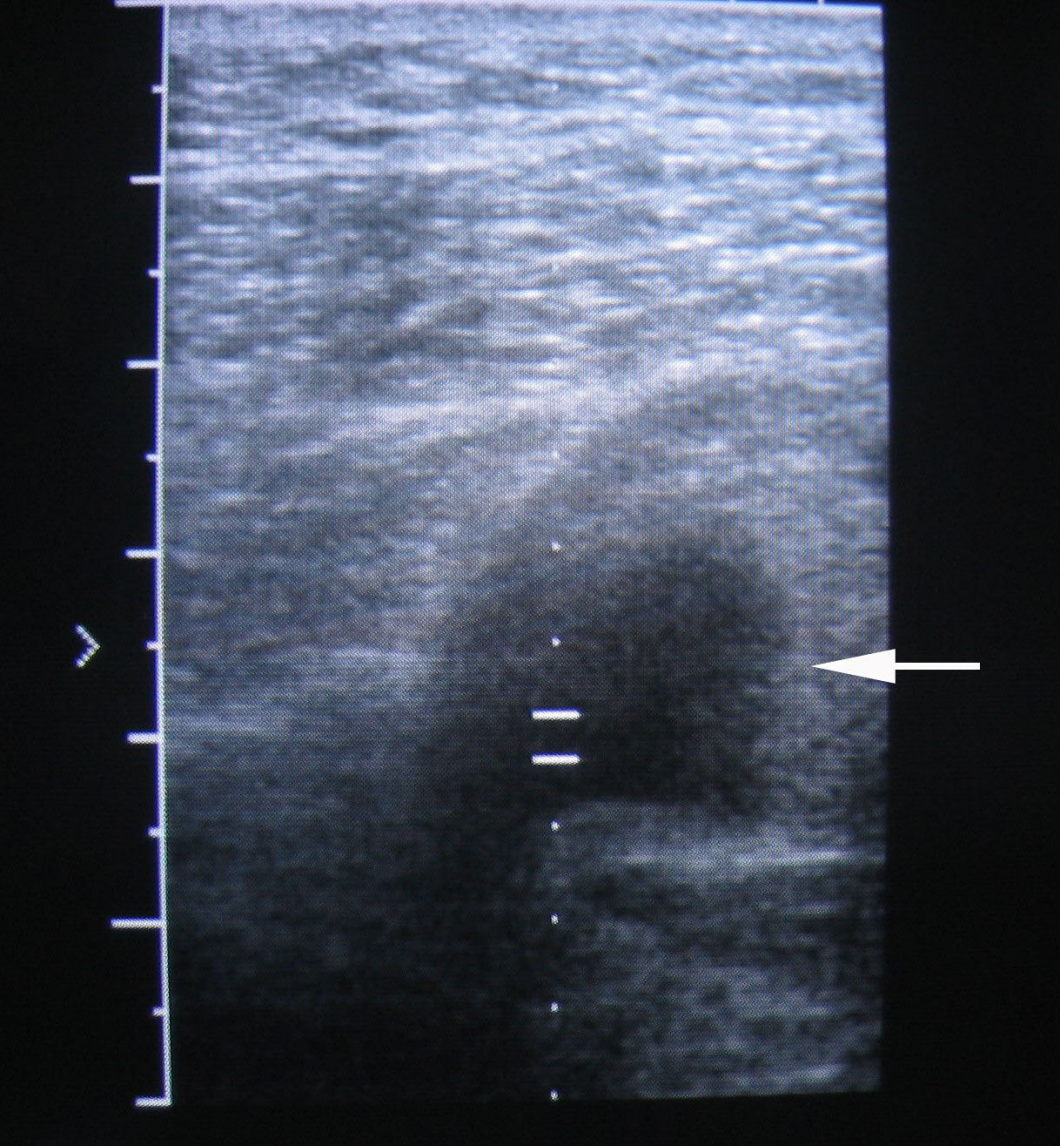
An ultrasound examination of his left buttock performed on the day of presentation showing an unexpected wide-diameter, pulsatile vascular structure with intra-luminal clot (arrow).
